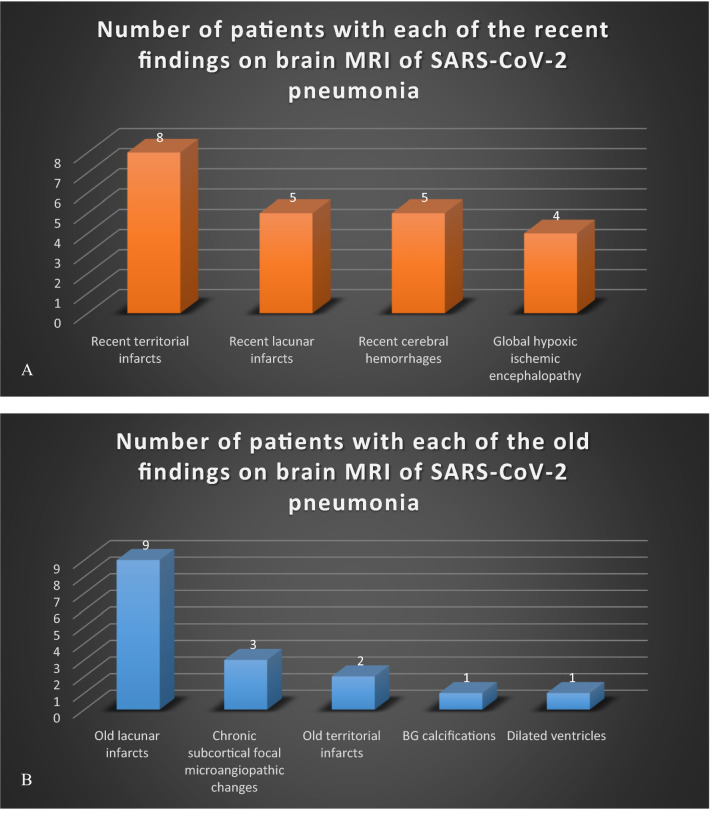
Frequency of patients with recent (A) and old (B) diagnostic findings on positive brain MRI of 38 among 46 patients with RT-PCR confirmed SARS-CoV-2 pneumonia and presented with neurological manifestations.
